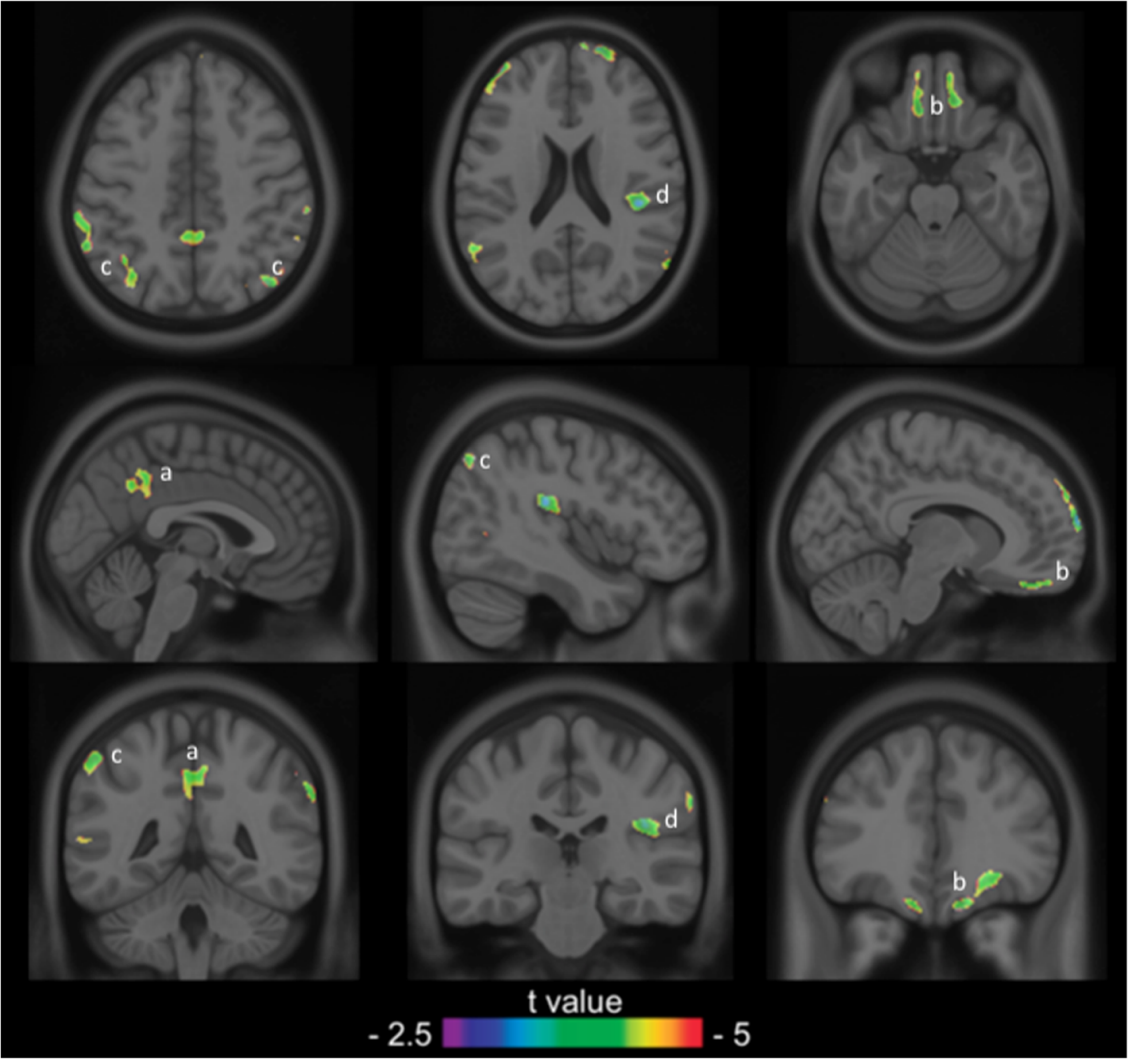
Higher NPI-Q and shorter EYO of AD were associated with higher [^18^F]FDG uptake decline in DIAD mutation carriers. Statistical parametric map overlaid on a structural MRI scan shows regions in the PCC (a), vmPFC (b), bilateral parietal lobes (c) and right AI (d) where higher [^18^F]FDG uptake decline was found in cognitively normal DIAD mutation carriers with higher NPI-Q scores and shorter EYO of AD. The analysis was corrected for gender, education, *APOE* ε4 status and family mutation type (*APP, PSEN1* and *PSEN2*) and multiple comparisons were corrected using random field theory at *p* <  0.001. AD: Alzheimer disease; AI: anterior insula; DIAD: dominantly inherited Alzheimer’s disease; EYO: estimated years to onset; [^18^F]FDG: [^18^F]fluorodeoxyglucose; NPI-Q: Neuropsychiatric Inventory; PCC: posterior cingulate cortex; vmPFC: ventromedial prefrontal cortex
